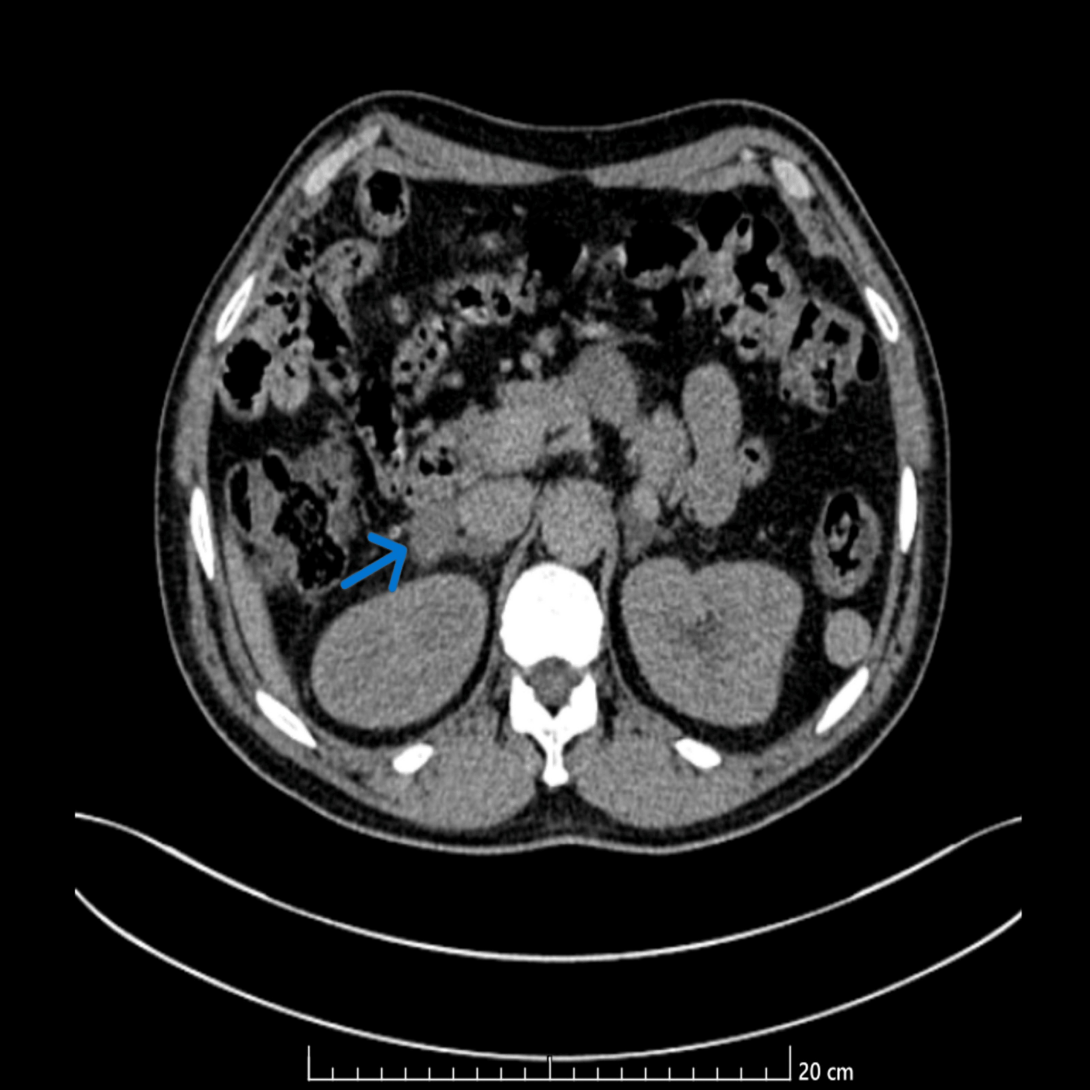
Axial contrast-enhanced abdominal CT scan demonstrating a right adrenal mass (blue arrow)
